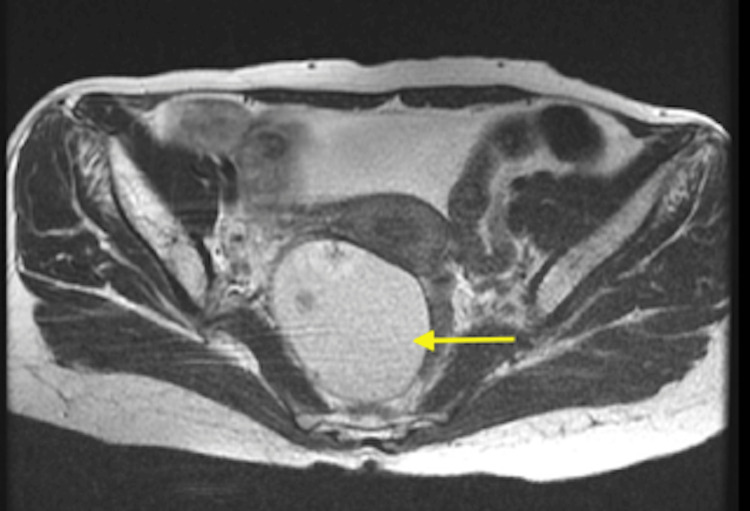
T2 MRI image of the patient The image shows a cystic pelvic-abdominal lesion of 15 x 12 x 11 cm marked with a yellow arrow MRI = Magnetic Resonance Imaging
